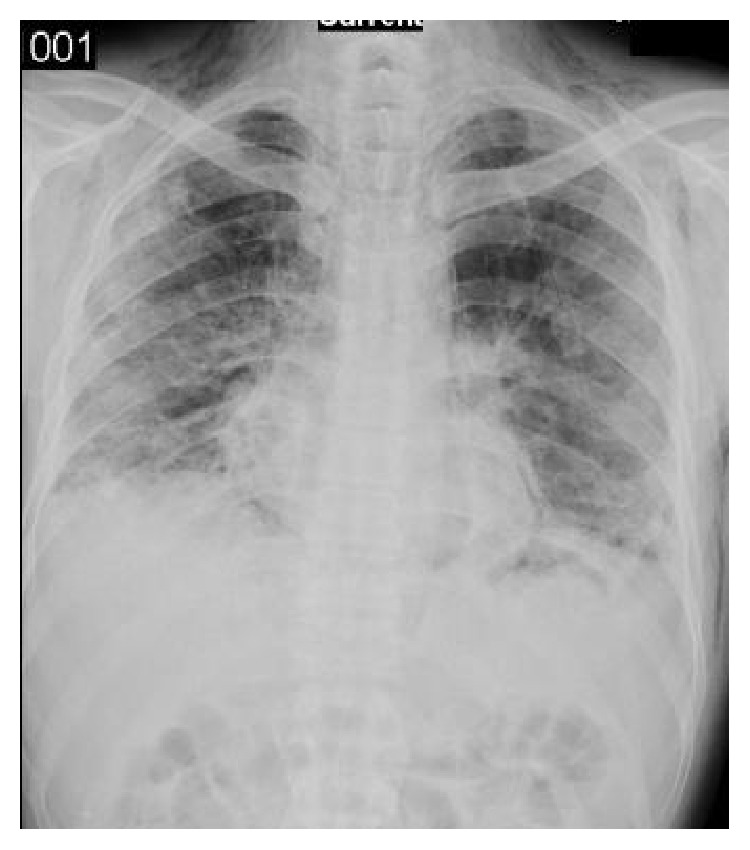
Chest radiograph showing bilateral opacities, pneumomediastinum, and subcutaneous emphysema.
